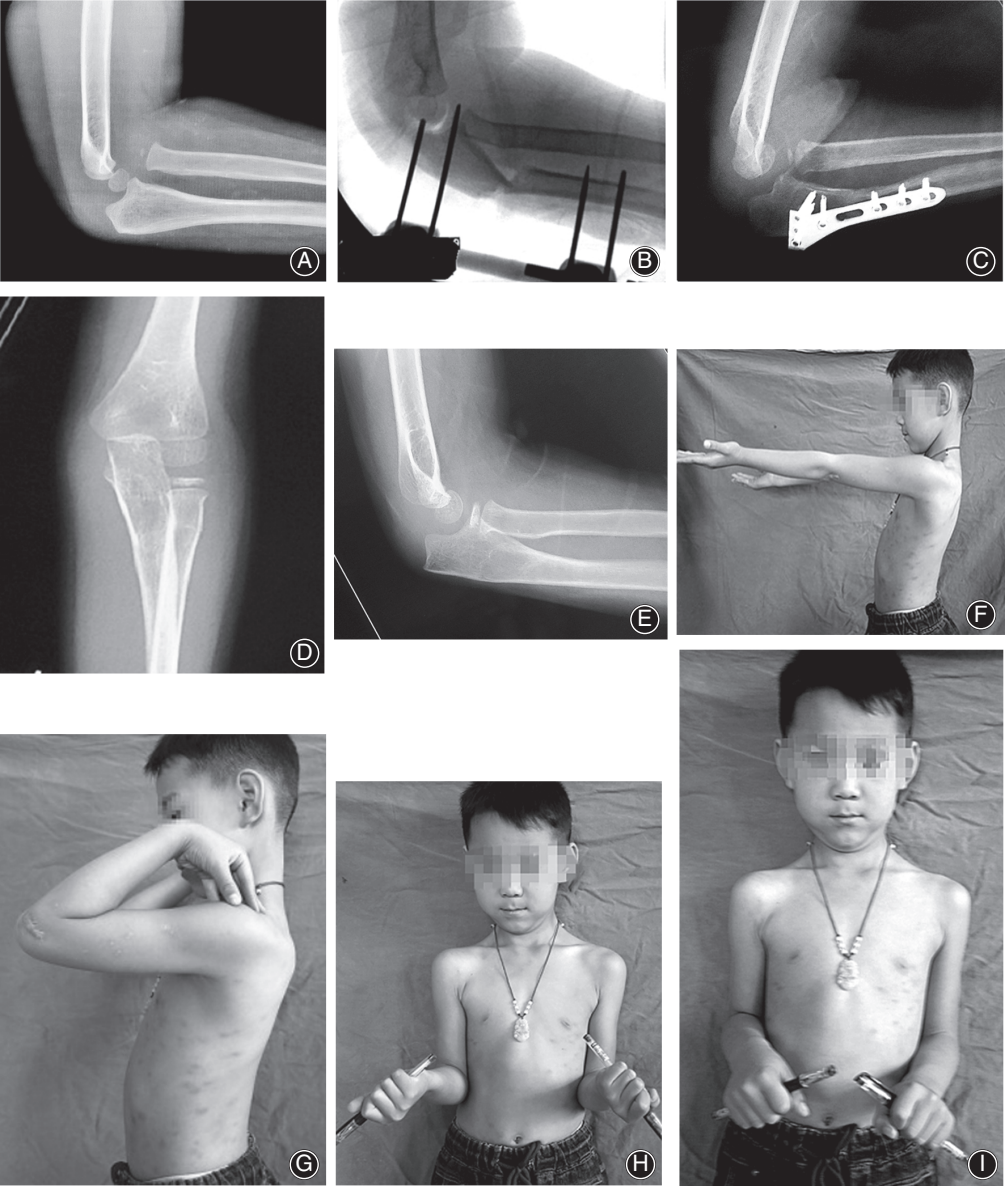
A 4‐year‐old boy fell to the ground and injured his left elbow 8 months before surgery. (A) Preoperative lateral radiograph of the elbow showing a missed Bado I Monteggia fracture. (B) Intraoperative fluoroscopic picture of ulna osteotomy with angulation and lengthening assisted by an external fixator. (C) Lateral radiograph of the elbow showing good remodeling at the osteotomy site at 6 months postoperatively before removing the plate. (D, E) Anteroposterior and lateral radiographs of the left elbow showing a well‐contained radial head at 3‐year follow‐up. (F–I) The clinical examination disclosing full range of motion of the elbow flexion (F), extension (G), supination (H), and pronation (I) at 3‐year follow‐up.
